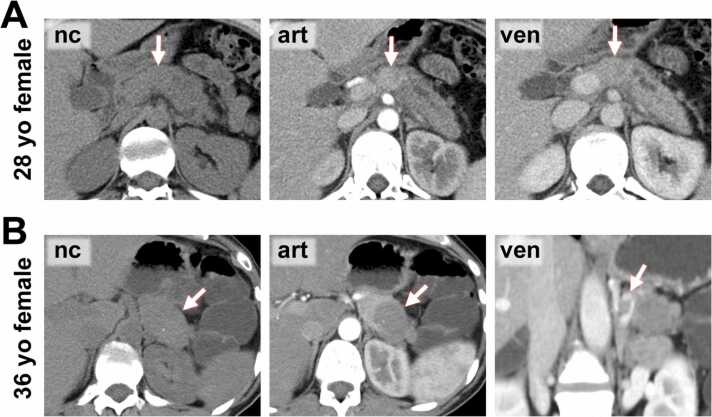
CT images from pNEN patients. C) 28-year-old female patient with a pNEN in the pancreatic body (*arrow*) which shows clear hyperdensity in the arterial phase and is associated with upstream dilatation of the main pancreatic duct. D) 36-year-old female patient with a pNEN with large cystic parts and a small calcification in the pancreatic tail (*arrow*). The encasement of the splenic vessels (depicted in the venous phase image) is helpful for differentiation from SPN.
